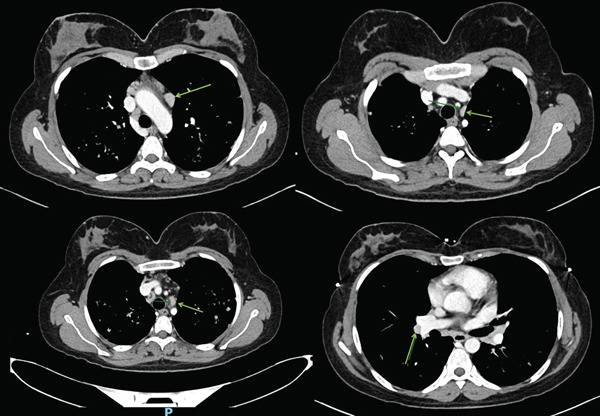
Axial mediastinal‐window chest CT images, demonstrating mild bilateral hilar and mediastinal lymphadenopathy.
